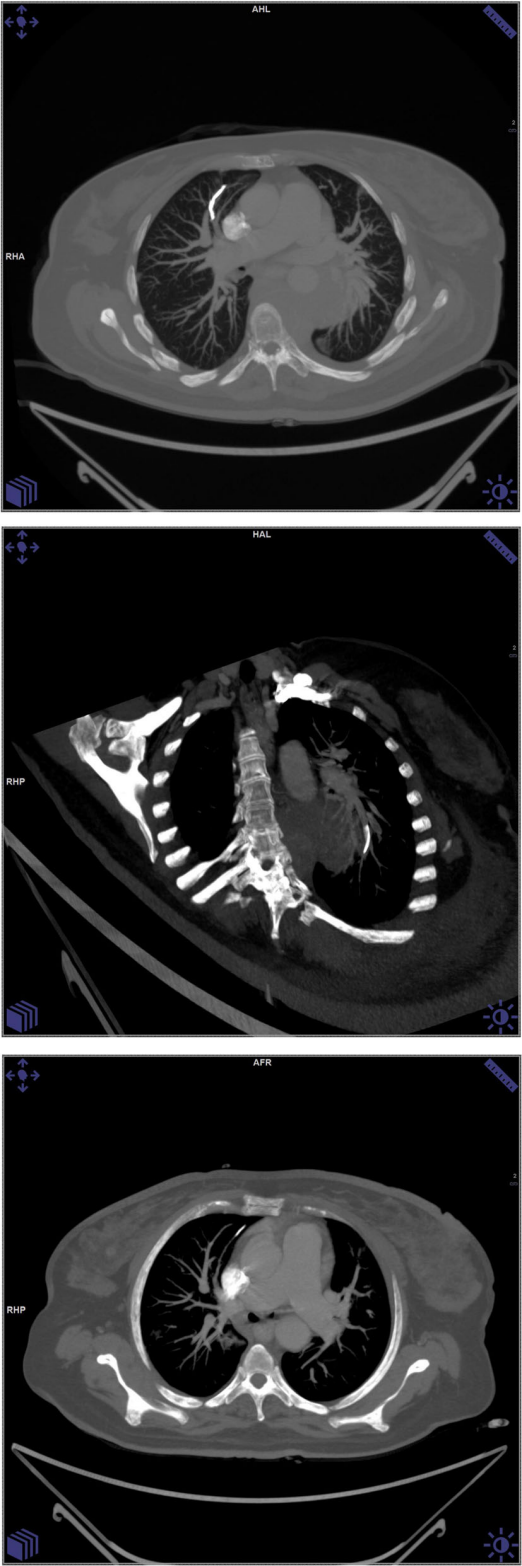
Chest CT with MIP showing a curvilinear density in the anterior subsegmental branch of Right Upper lobe indicating cement PE. Oblique coronal MIP image of the same patient showing cement PE in basal subsegmental branch of LLL
